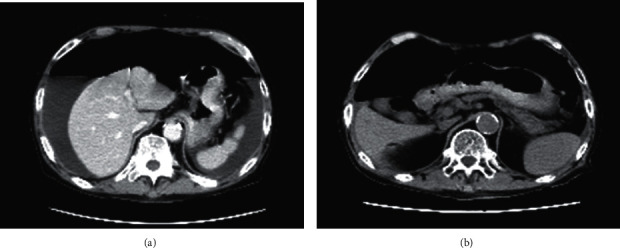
(a), (b) Computed tomography of the abdomen one day after admission. Marked pneumoperitoneum was observed.
